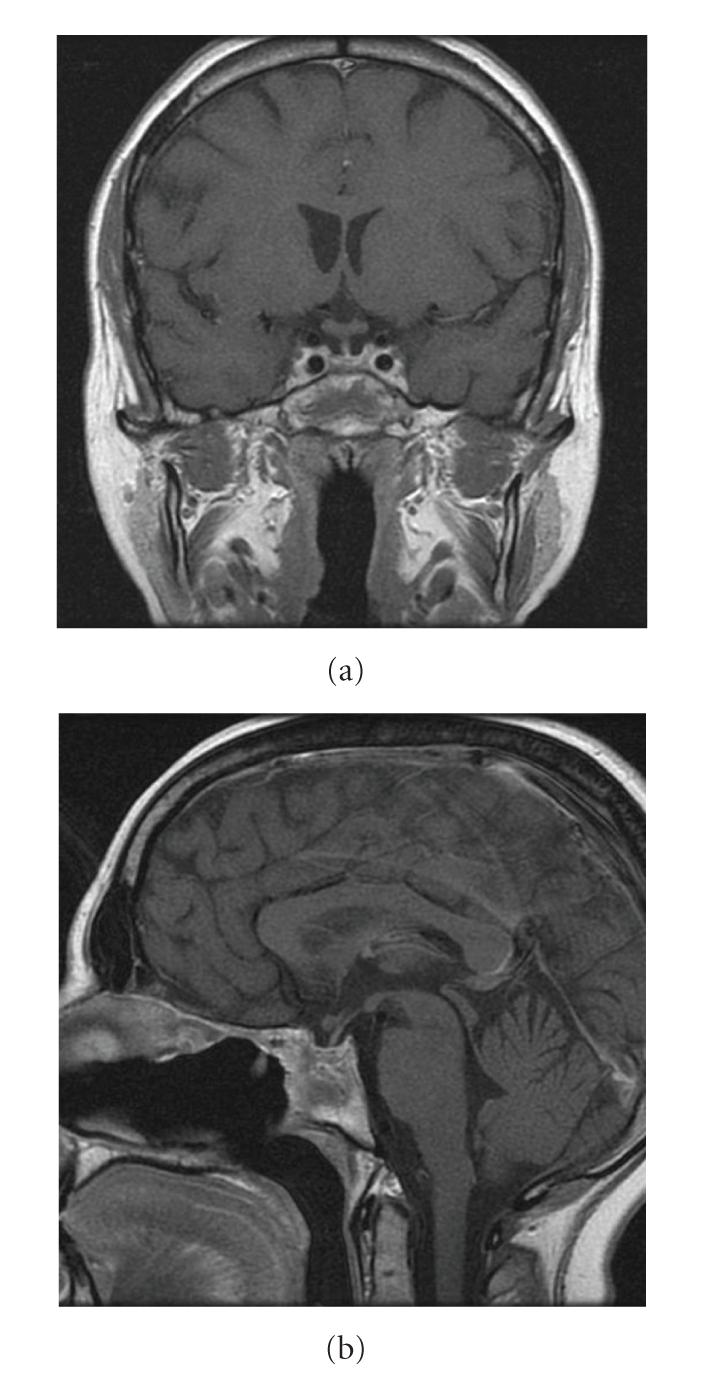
Postoperative coronal (a) and sagittal (b) T1-w MR images, showing no pathological changes in the sellar region.
